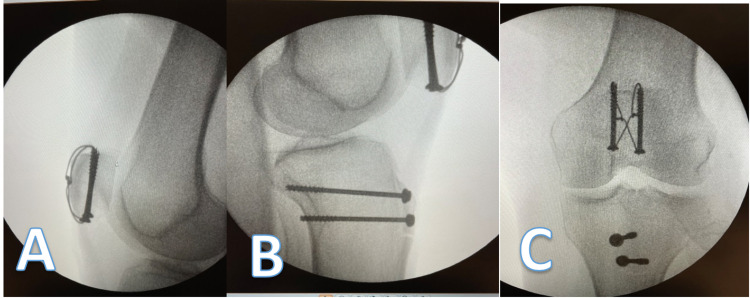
Lateral (A,B) and anterior-posterior (C) radiographs taken intra-operatively.
